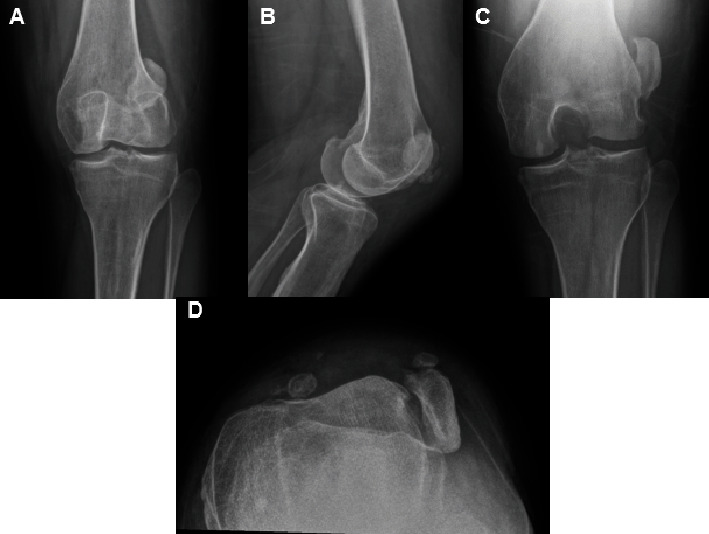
Preoperative radiographs. Preoperative radiographs of the patient's left knee done at an outside clinic. (A) Anteroposterior, (B) lateral, (C) tunnel, and (D) merchant radiographs demonstrating a complete lateral dislocation of the patient's left patella with associated large fracture fragment in dysplastic femoral trochlea with a chronic type avulsion calcification off the medial patella.
